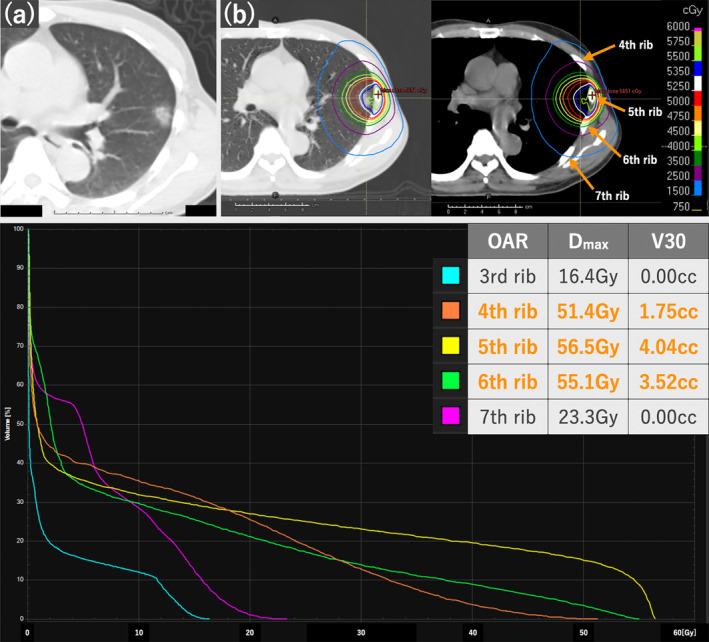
(a) Chest computed tomography (CT) scan showing a tumor in the left upper lobe adjacent to the chest wall. (b) Stereotactic body radiotherapy (SBRT) was performed with 48 Gy in four fractions; isodose lines of 100% (red), 50% (purple), and 30% (cobalt) are shown. Dose‐volume histogram of each rib; third rib (light blue), fourth rib (orange), fifth rib (yellow), sixth rib (light green), and seventh rib (pink).
